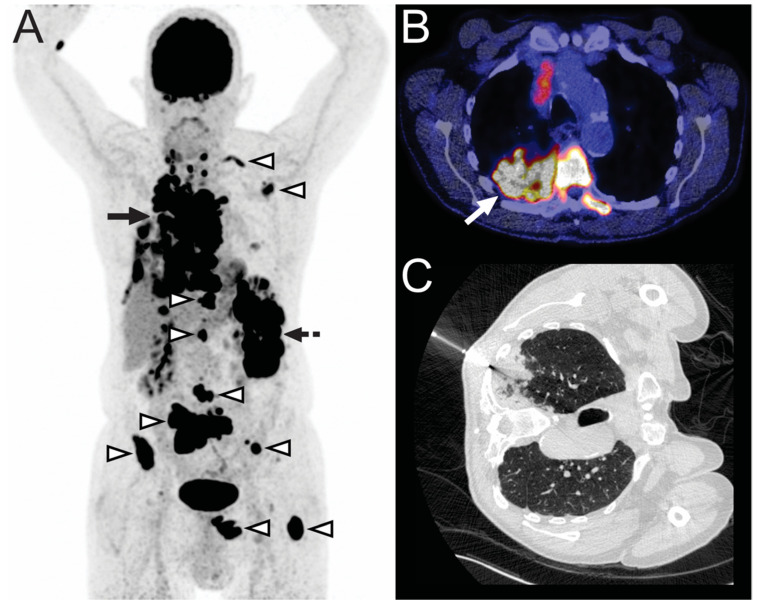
A 78-year-old man with a lesion in the right upper lobe, suspicious for lung cancer, was treated with radiation therapy, although no pathologic diagnosis was made. Two months later, a CT scan (not shown) suggested tumor progression. Subsequently, he was referred to our tertiary care center. FDG-PET (A) showed an FDG-avid mass in the right lung (continuous arrow), FDG-avid lesions in the spleen (dashed arrow), and multiple FDG-avid bone lesions (arrowheads), suspicious for metastatic disease. The mass in the right lung is also shown on fused FDG-PET/CT ((B), arrow). CT-guided biopsy was ordered by the pulmonary oncologist for lung cancer mutation analysis. CT image (C) shows the biopsy needle just before tissue sampling of the mass in the right lung. Pathologic examination revealed diffuse large B-cell lymphoma and no lung cancer.
